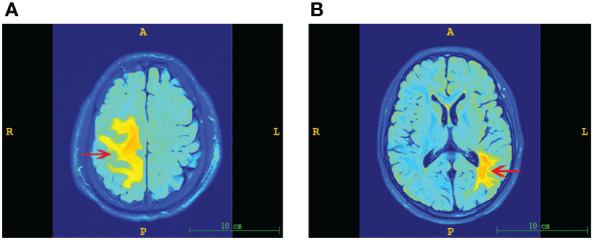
Representative MR images of cerebral lesions of varying size in PACNS patients. Dominant lesions are marked by arrows. (A) Image of a larger lesion showing hyperintense regions on FLAIR imaging. (B) Image of a smaller lesion observed on FLAIR imaging.
